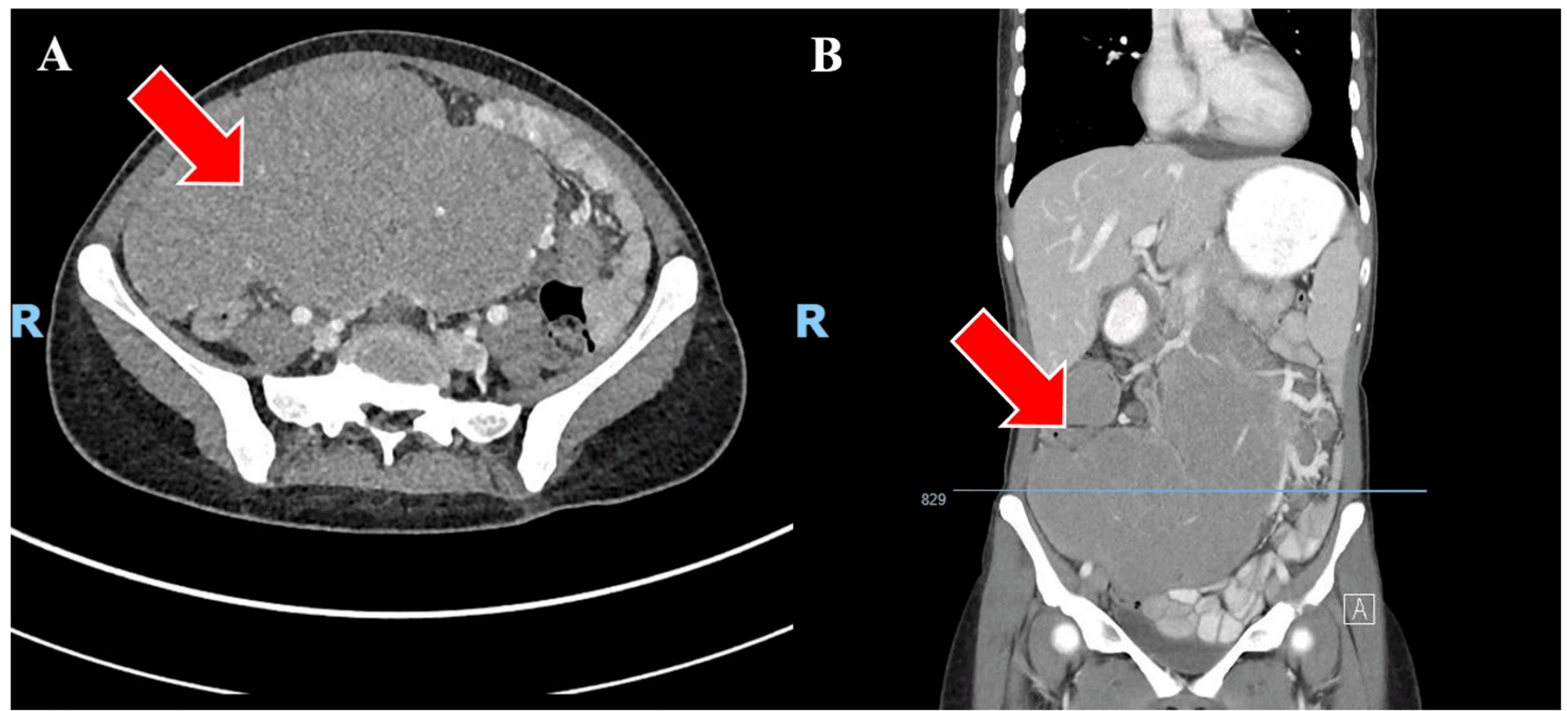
Axial (A) and coronal (B) contrast-enhanced CT scans of the abdomen and pelvis demonstrating a 19 x 18 x 9 cm mass (red arrow). It encases branches of the superior mesenteric artery and there is abnormal configuration of the ileo-caecal valve in the right side of the abdomen.
